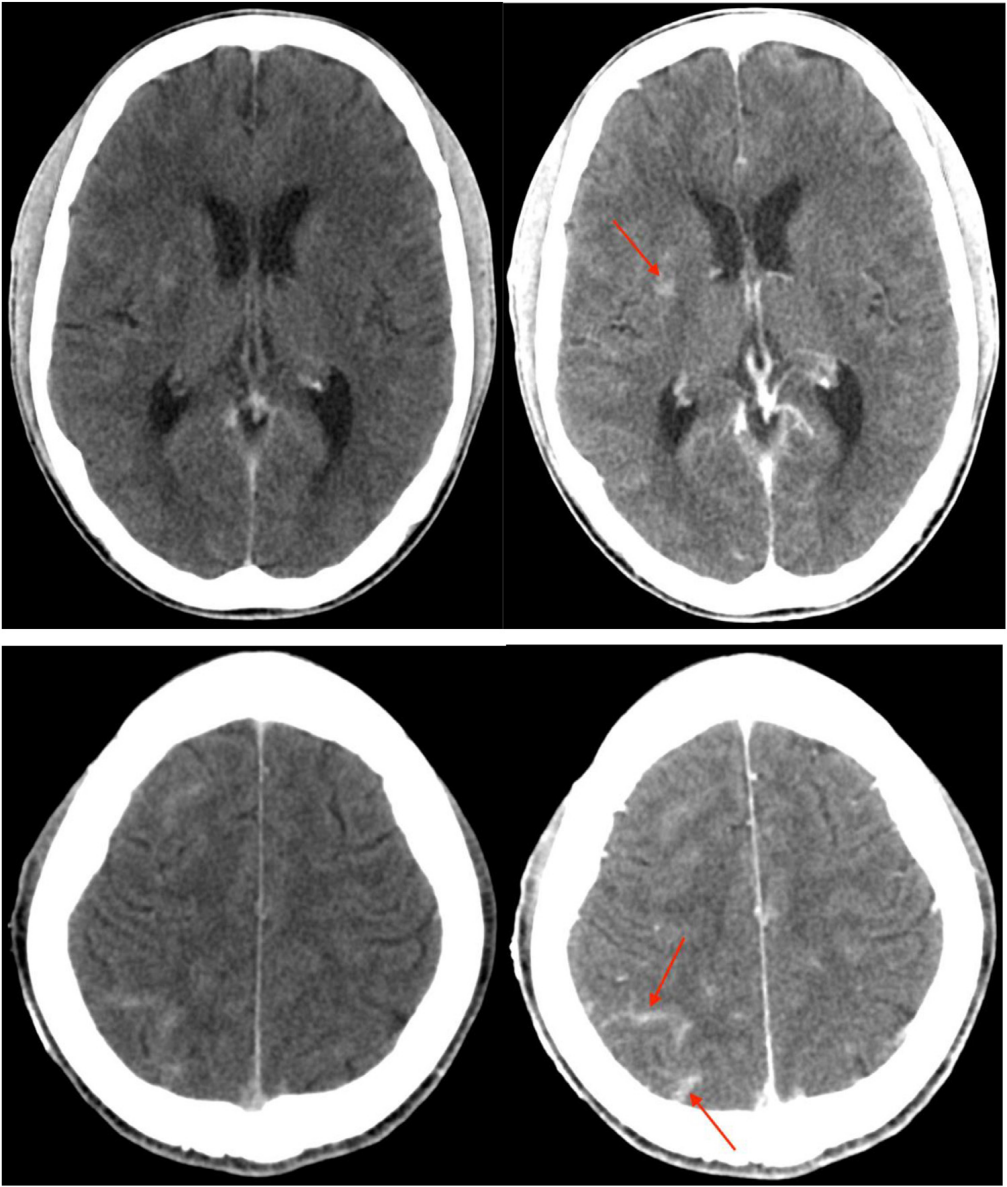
Axial pre (A) and postcontrast (B) CT images demonstrate small regions of enhancement within the basal ganglia, more conspicuous on the right (red arrow). On a lower slice of the brain, axial pre (C) and postcontrast (D) images demonstrate sulcal hyperdensity, most pronounced within the right parietal lobe (red arrows), and likely relating to leptomeningeal enhancement.
